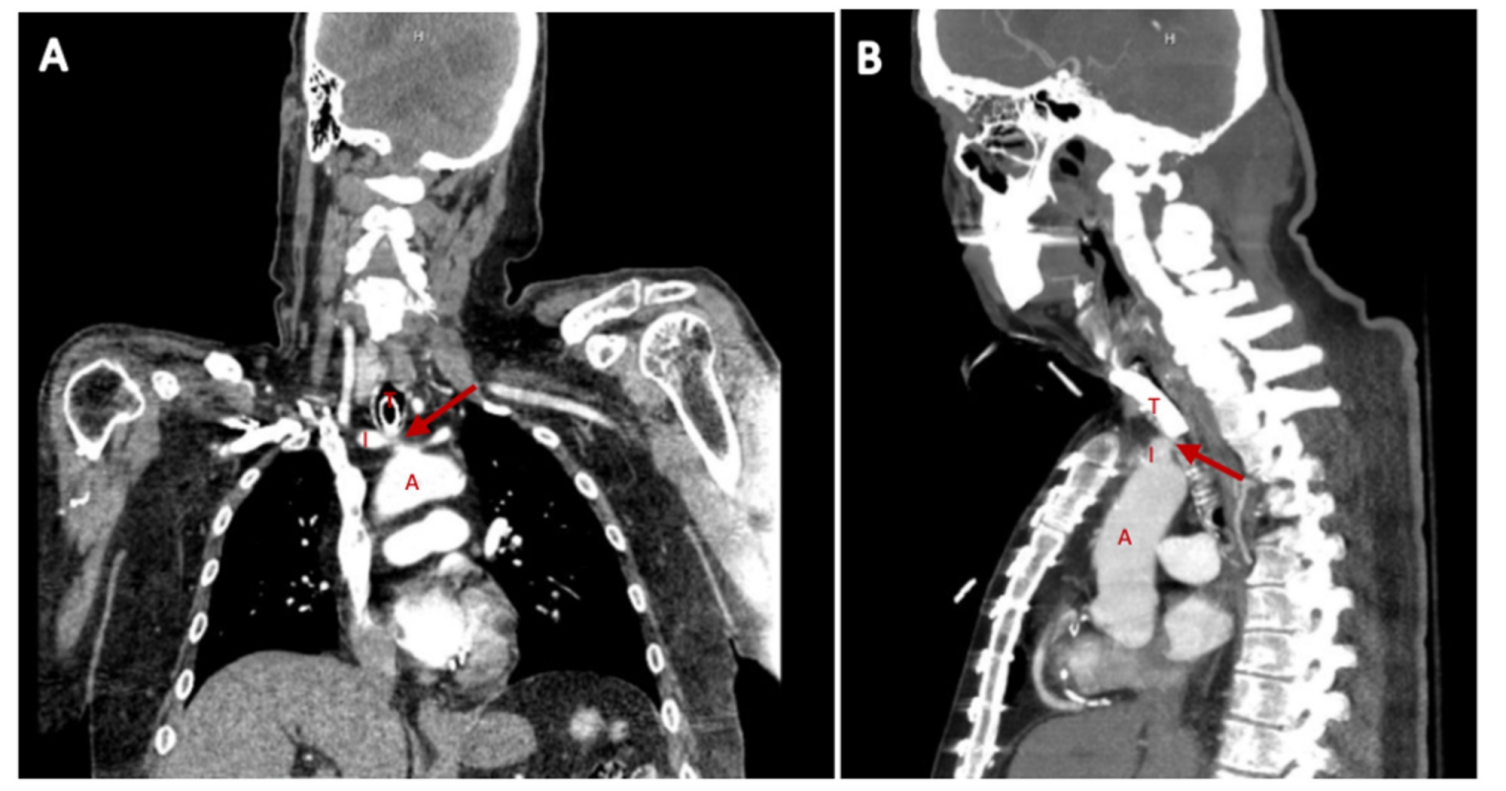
Coronal (A) and sagittal (B) views of CT angiogram of the chest and neck revealing a tracheoinnominate artery fistula (arrows). I: innominate artery, T: trachea with tracheostomy tube, A: aortic arch.
